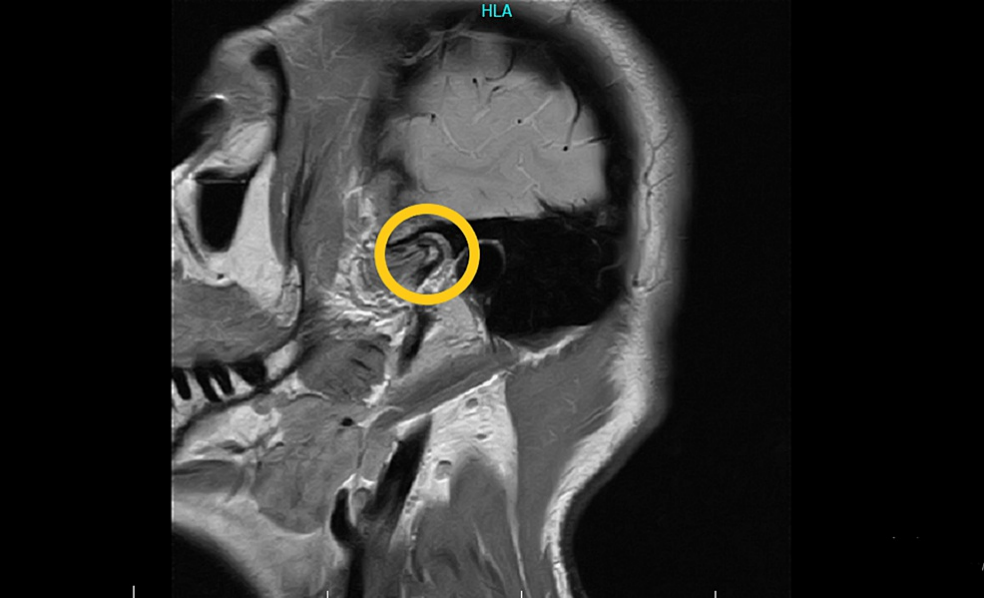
MRI of the patient's right temporomandibular joint one year after stem cell therapy MRI: Magnetic resonance imaging
